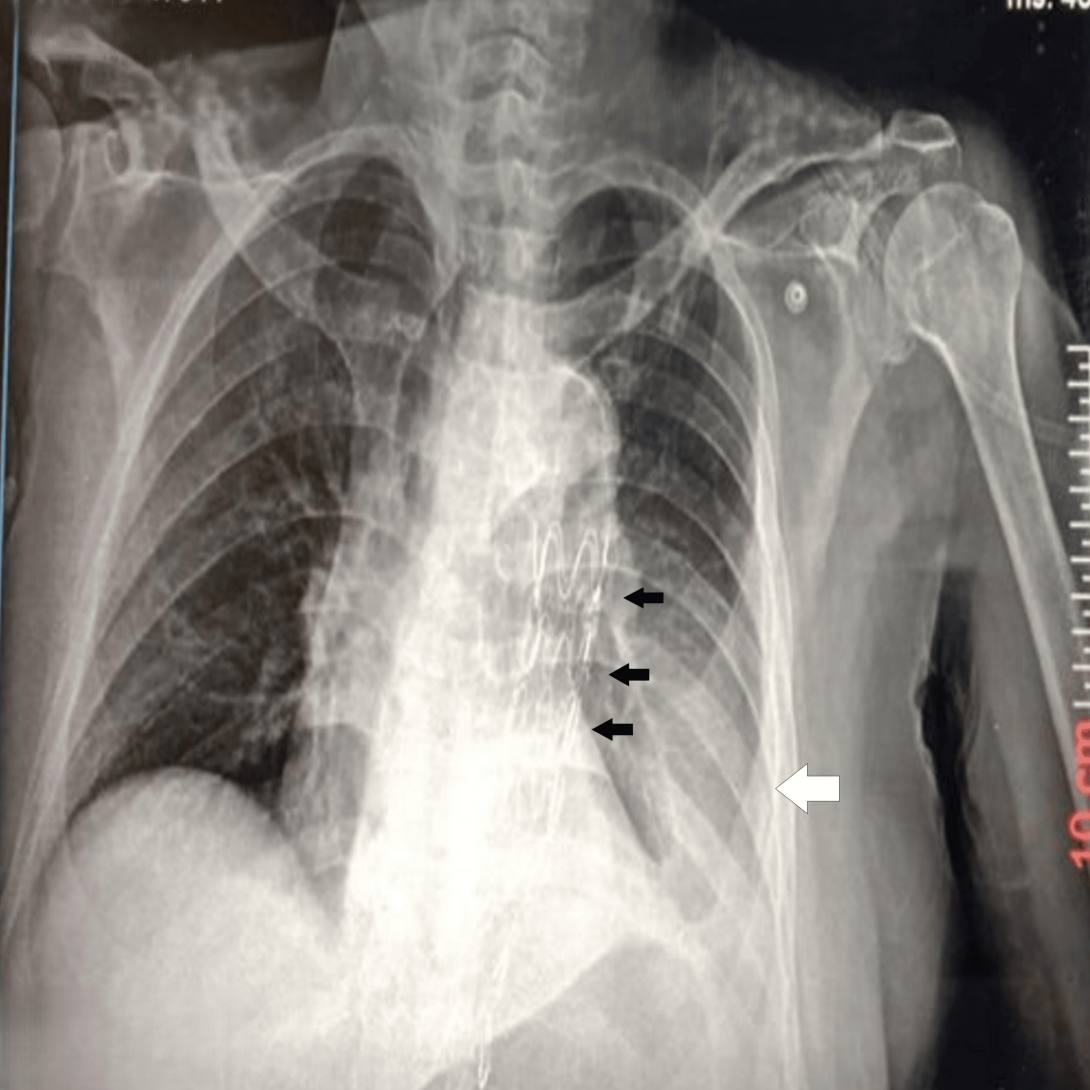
Repeat chest X-ray after 48 hours of procedure showing improvement of lung opacities (pointed by a white arrow) and stent graft in descending thoracic aorta (pointed by black arrows).
